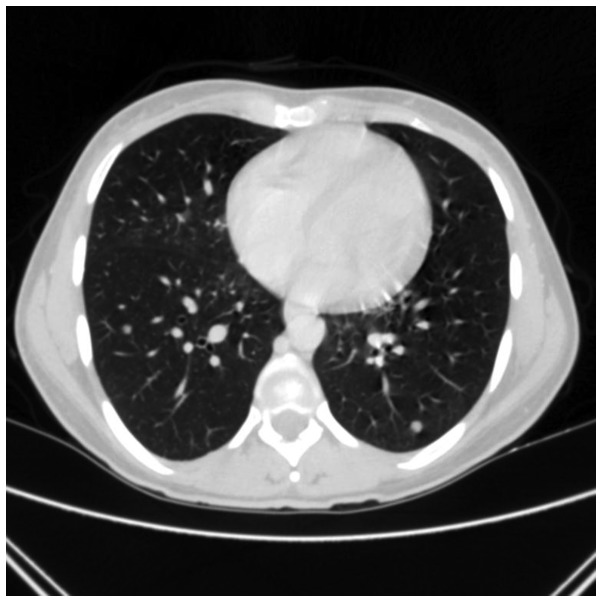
Computed tomography scan of the chest in axial section showing metastatic lesions.
